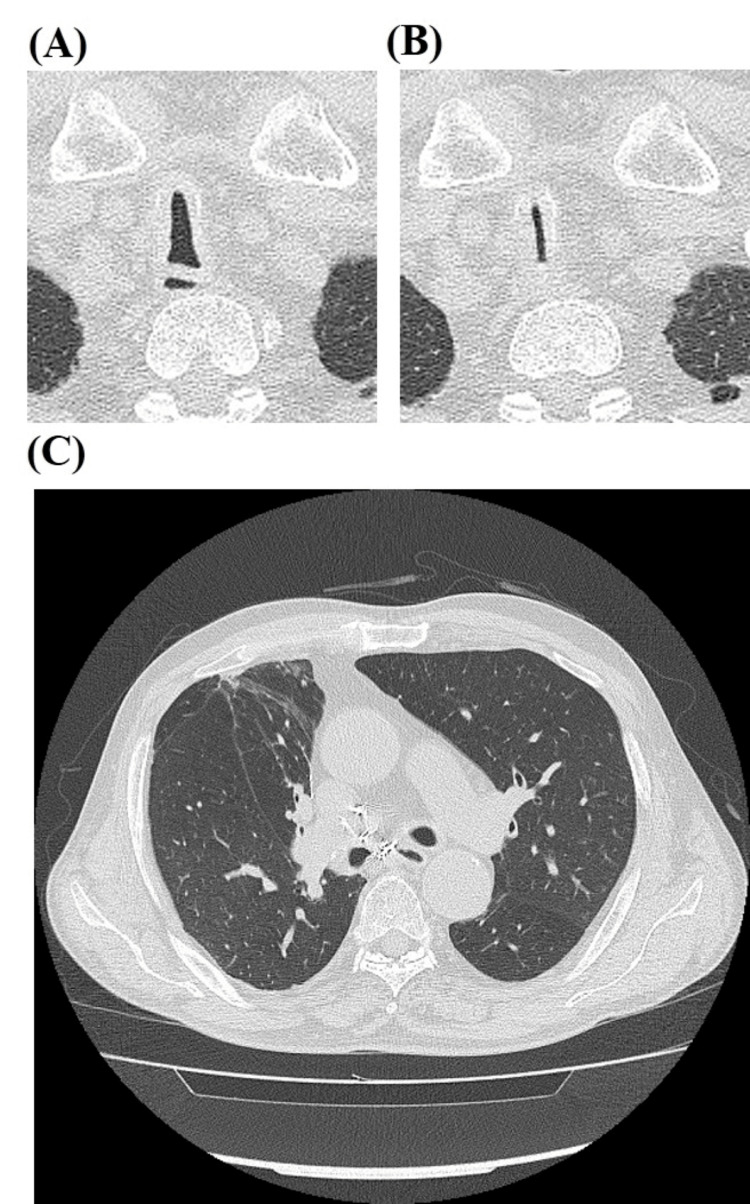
Chest computed tomography (CT) in the inspiration (A) and expiration (B) phases showing the saber-sheath trachea 1 cm above the aortic arch. (C) High-resolution CT demonstrating centrilobular nodules and bronchiolar wall thickening in the peripheral airways.
